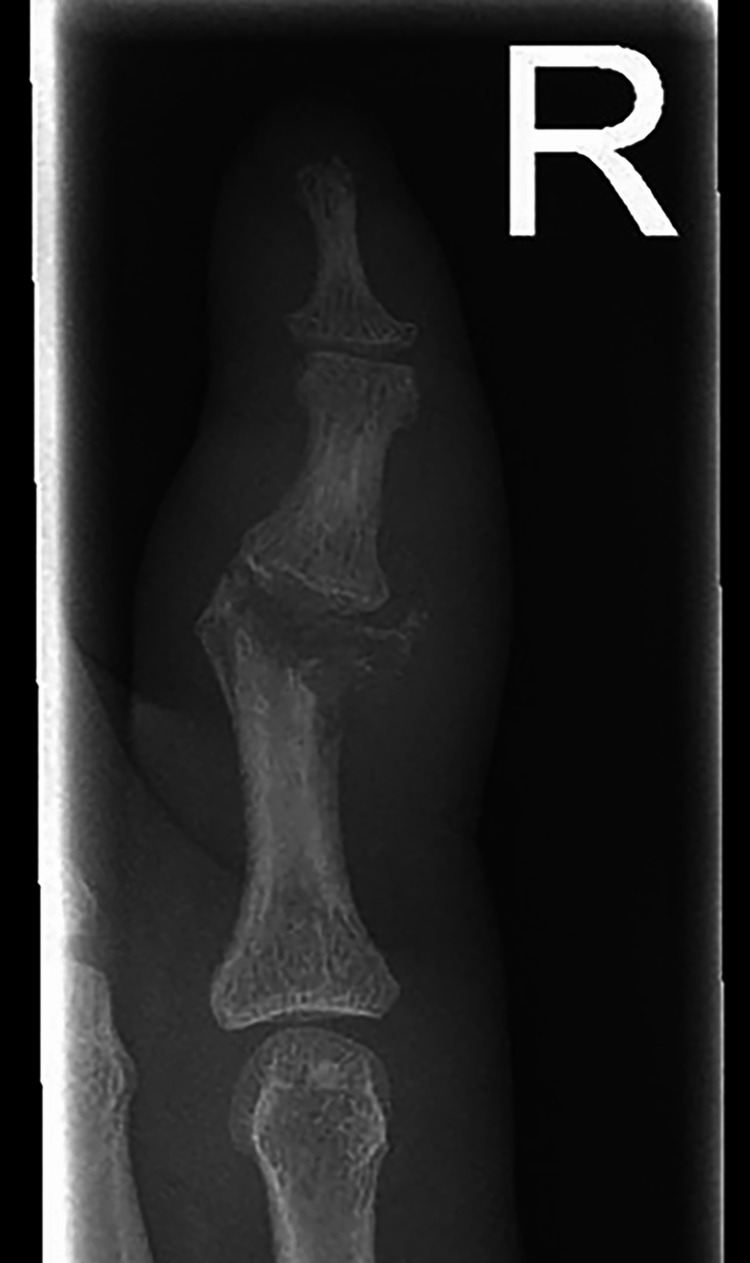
Plain radiographs showed RSF-PIP joint destruction with osteolysis and an increased soft tissue shadow, AP view. RSF: right small finger, PIP: proximal interphalangeal, AP: anteroposterior view.
